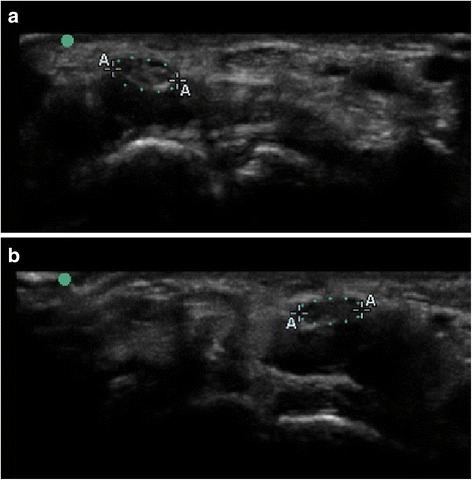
Ultrasound transverse view of the patient’s wrist. a Left wrist (the palm of which suffered from numbness and tingling). b Right wrist. Dashed circles indicate the median nerves. The figure shows enlargement of the left median nerve compared to the right median nerve
